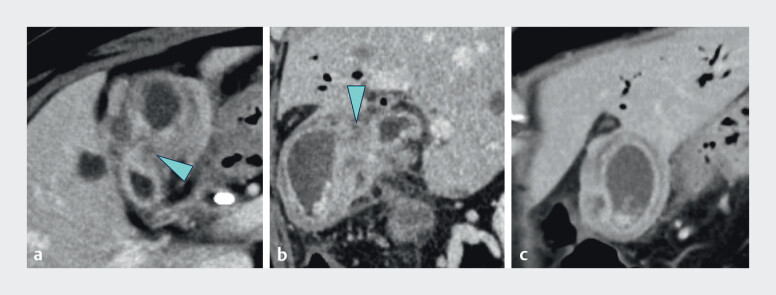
Computed tomography showing a ventrally located stricture in the gallbladder body due to adenomyomatosis (arrowhead) and localized cholecystitis confined to the fundus:
